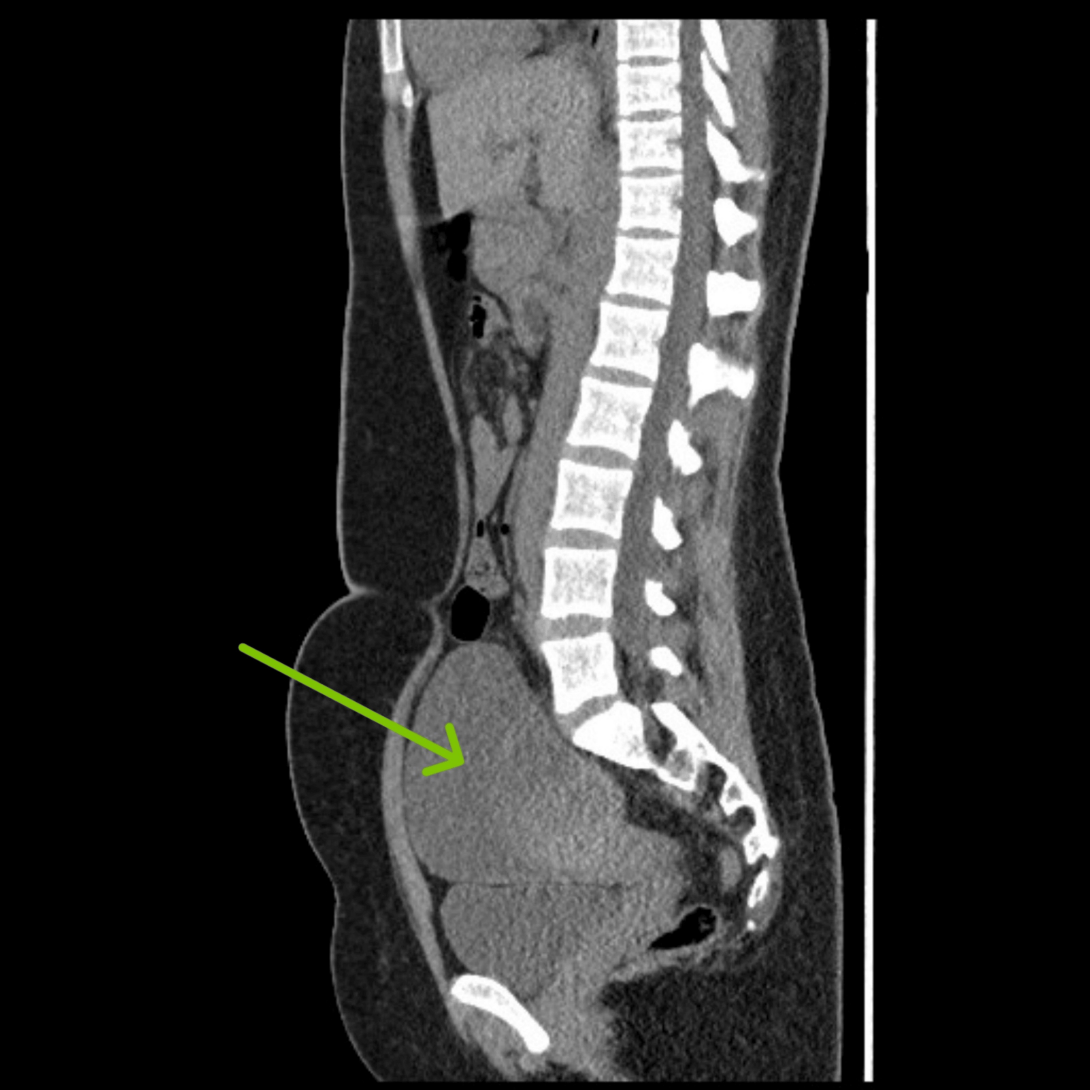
CT abdomen and pelvis, sagittal view. The arrow points to the enormous left adnexal cyst.
